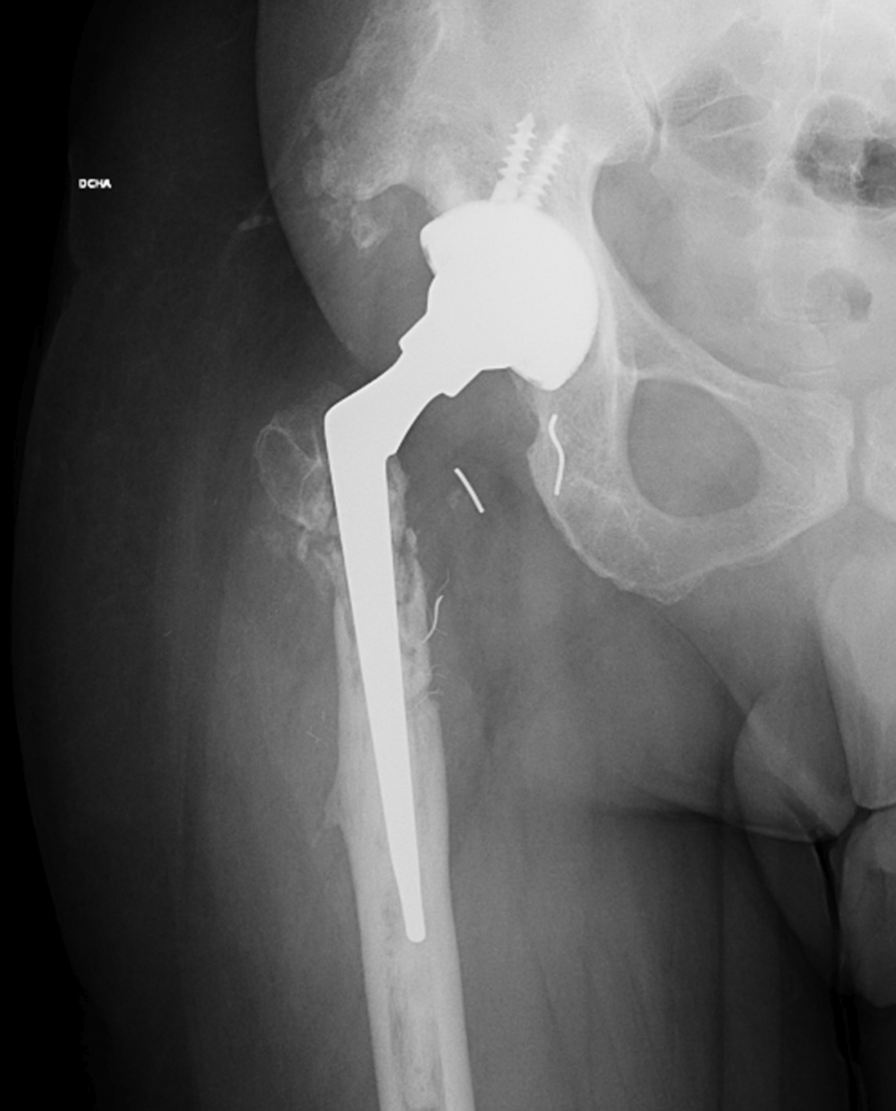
Postoperative anteroposterior radiograph of the hip after the second revision.
